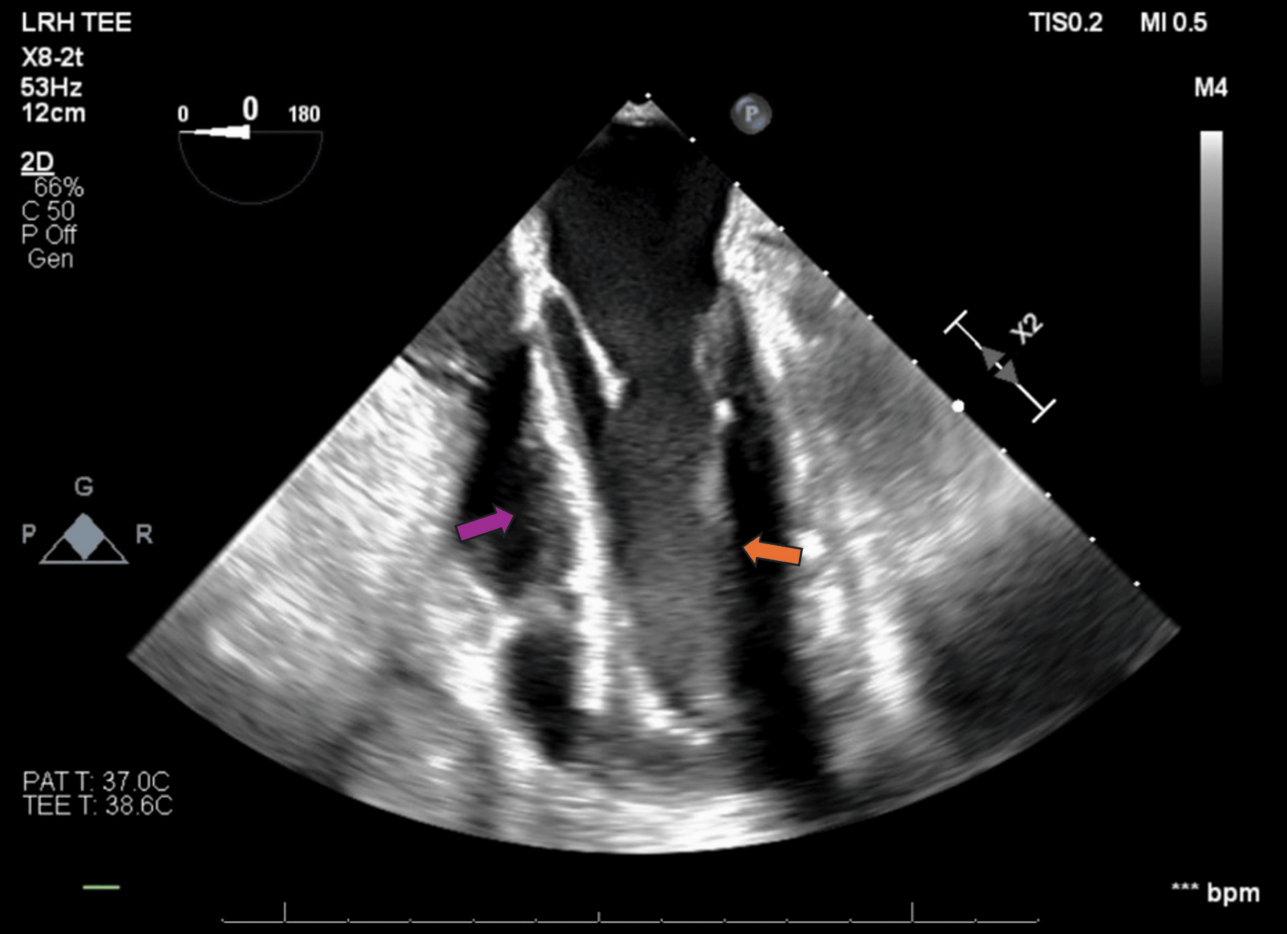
Transesophageal echocardiogram pre-pericardiectomy (mid-esophageal four-chamber view) The image reveals compression of both the right and left ventricles. The purple arrow indicates the compression of the right ventricle, while the orange arrow indicates the compression of the left ventricle.
